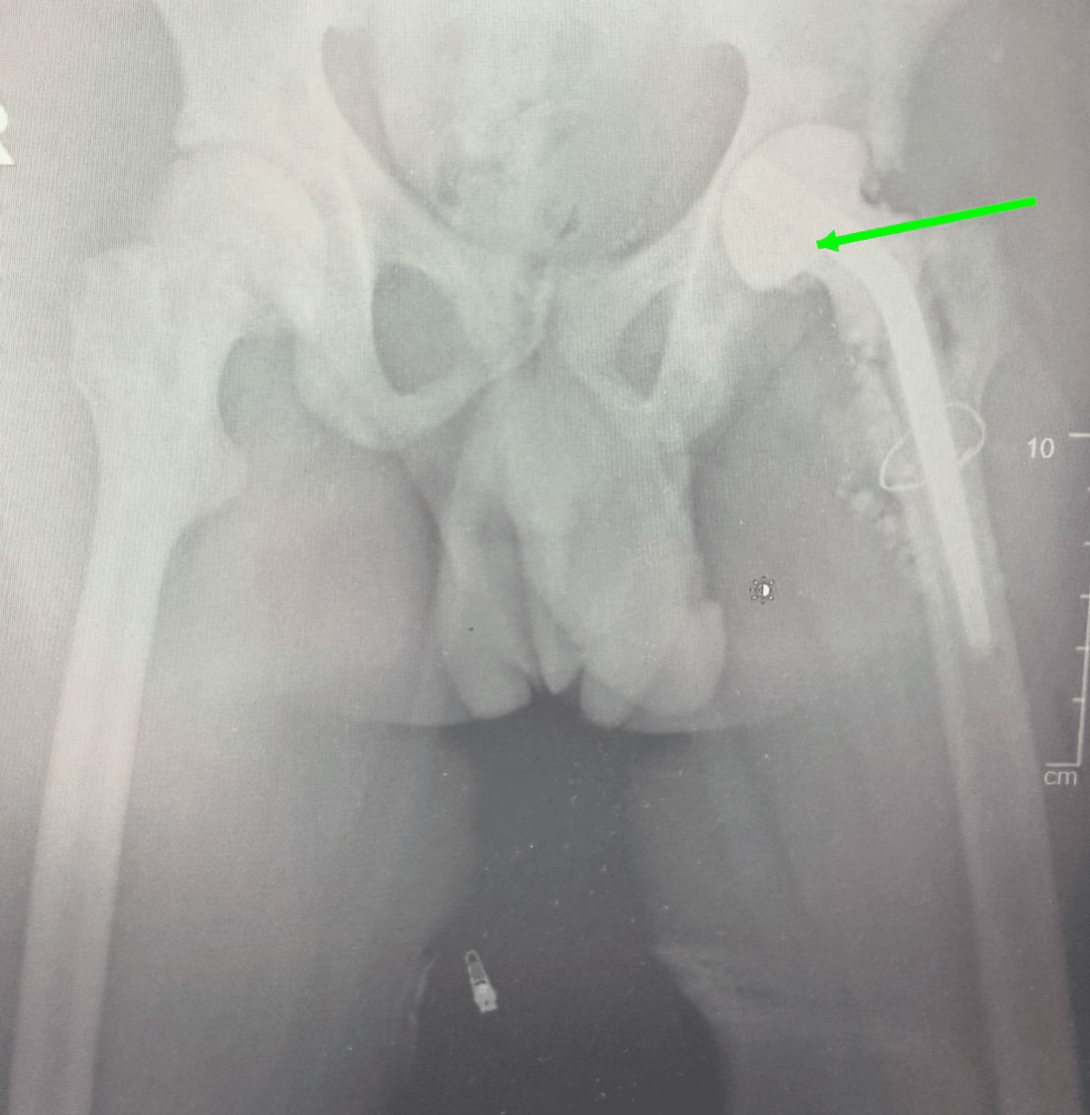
Cement spacer following first-stage THA Immediate postoperative X-ray of the pelvis following first-stage THA showing antibiotic loaded cement spacer implantation (green arrow). THA: total hip arthroplasty.
